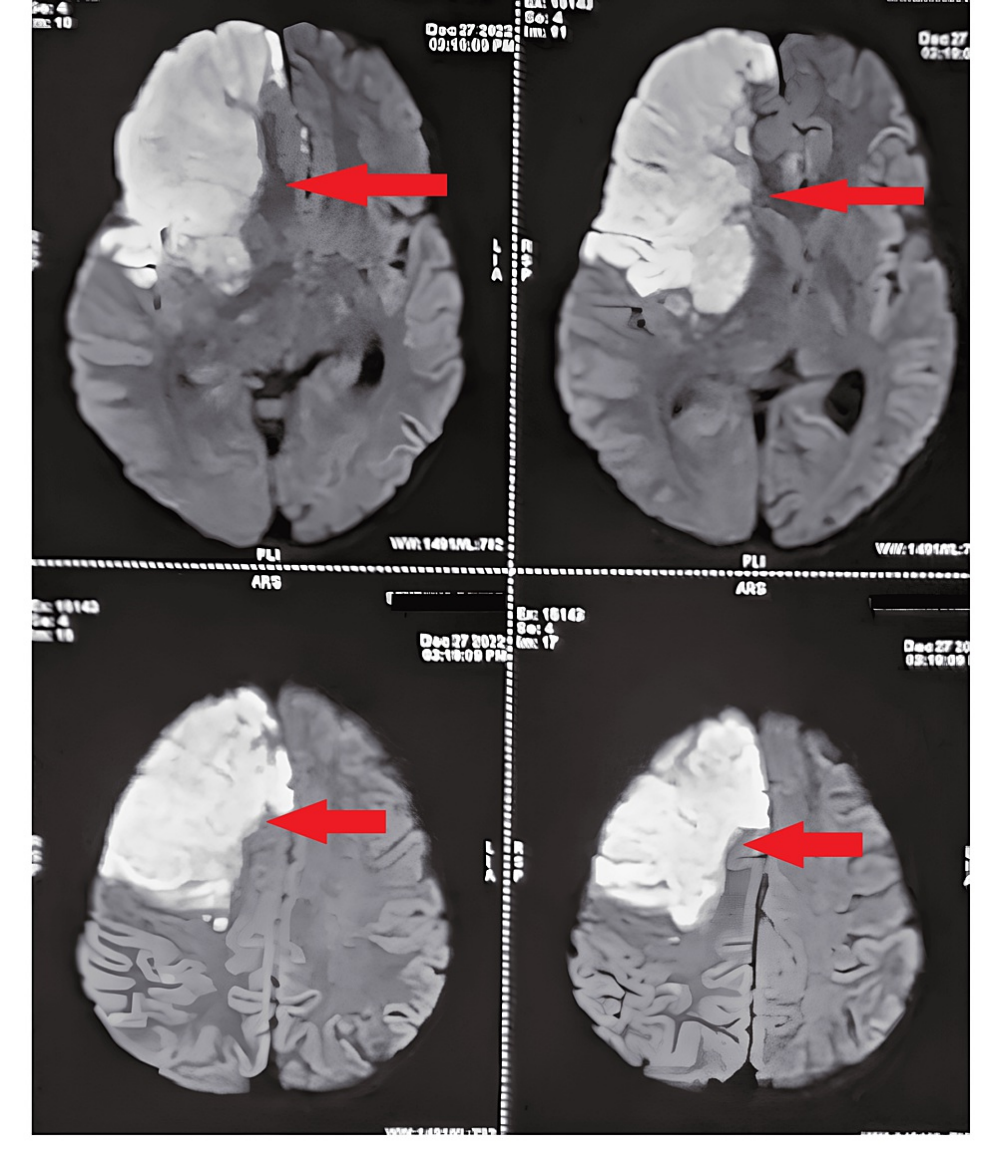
Magnetic resonance imaging (MRI) shows the infarct The red arrow shows the infarct in the brain.
